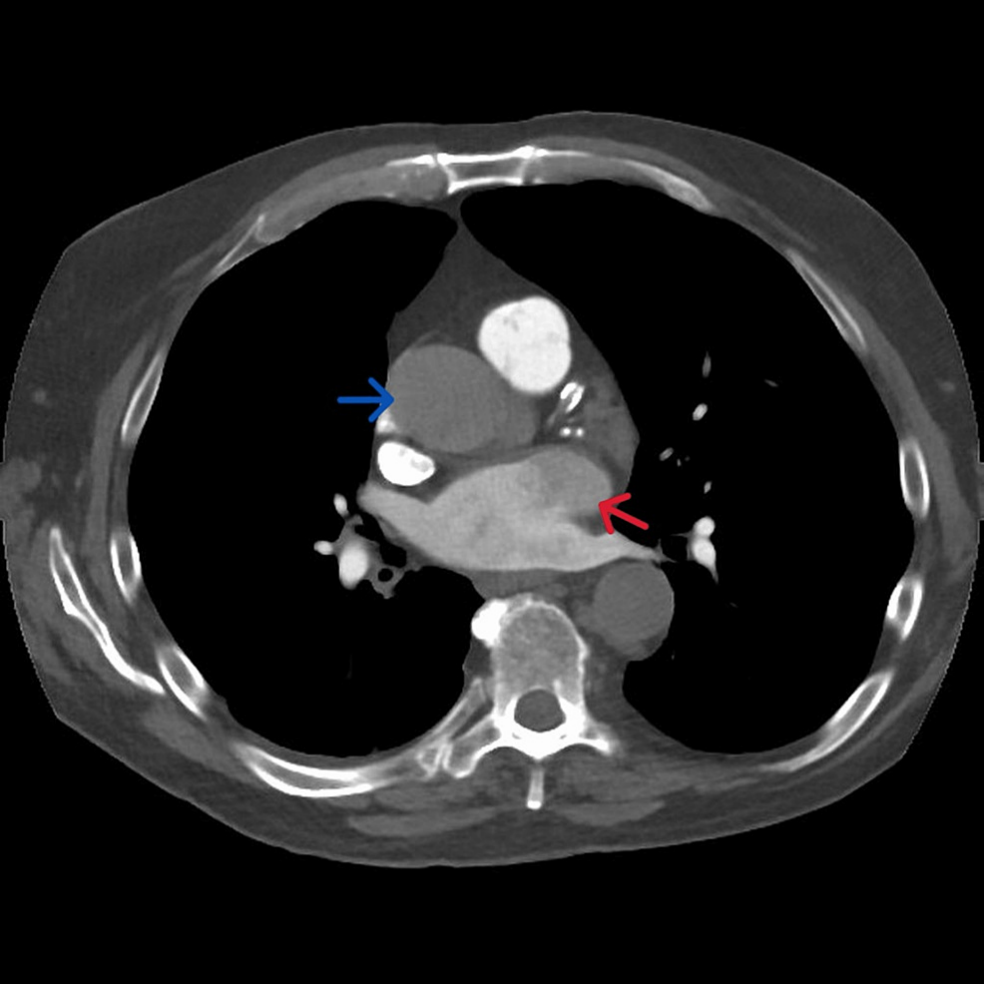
Thrombus in the right atrium and vena cava seen on CT (red arrow: thrombus in vena cava; blue arrow: thrombus in right atrium)
